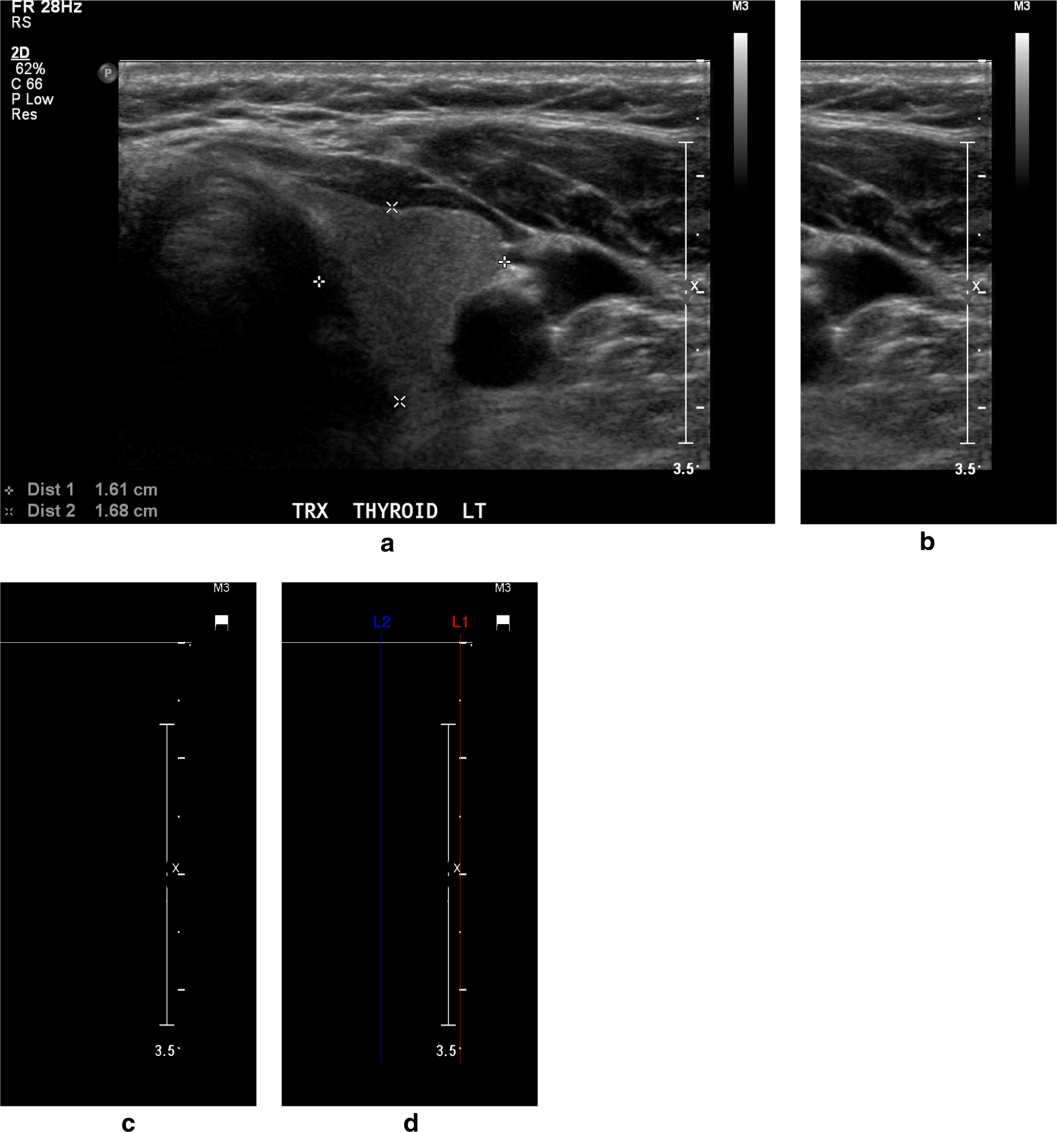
Detection of pixels deemed to be ticks by intensity thresholding. a Input thyroid ultrasound image. b Thirty percent right region of the input image where the thresholding was applied. c Binary image showing the pixels deemed likely to be ticks, and *red line* in d represents the column containing the tick bar
